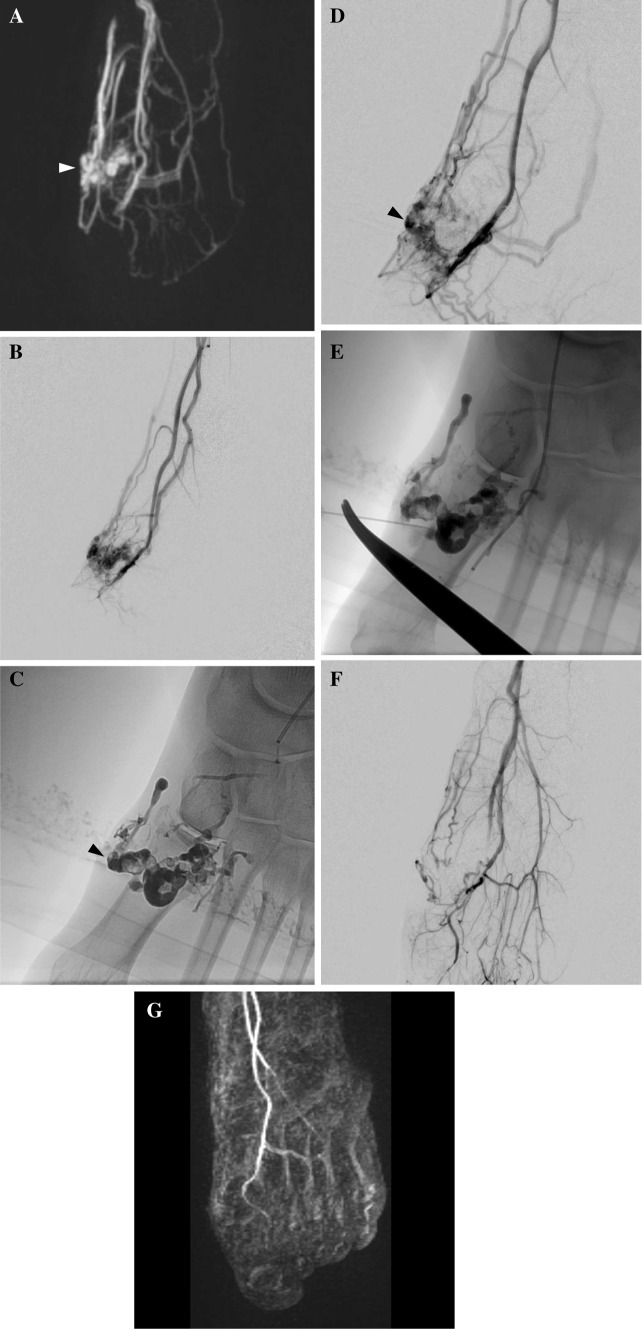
27-year-old woman with type II AVM of the left foot sole with worsening swelling (Patient No. 20). A Maximum intensity projection (MIP) image of coronal contrast-enhanced MR angiography shows an AVM (arrowhead) with dilated feeding arteries and draining veins in the left foot internal sole. B Angiography demonstrates an AVM with multiple feeders arising from dorsal artery and plantar arteries and draining veins. C Radiography showing NBCA/lipiodol casts (arrowhead) after transarterial embolization of 2 feeders. D Angiography after NBCA embolization shows reduced flow of AV shunts (arrowhead). E Remaining AV shunts were punctured using a 24 gaugeneedle, and sclerotherapy was performed by injecting polidocanol mixed with contrast medium under proximal compression using a tourniquet. F Angiography after embolization and sclerotherapy shows complete exclusion of AVM. G Contrast-enhanced MR angiography eighteen months after treatment shows complete exclusion of the AVM. The patient has had no recurrence, and the symptom has resolved. The therapeutic outcome was PR
